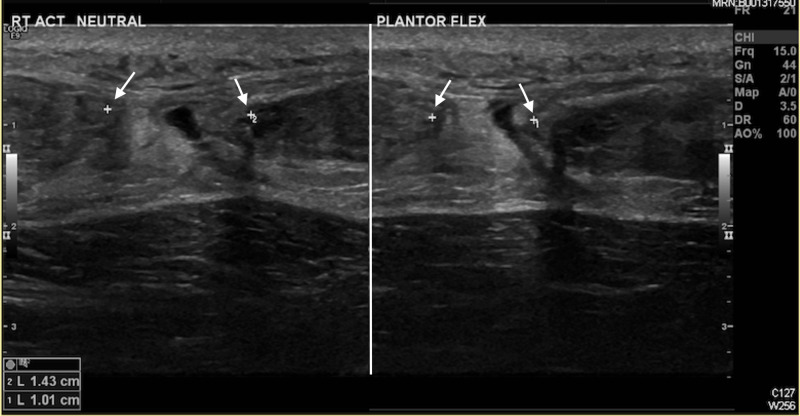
Ultrasound scan of a ruptured Achilles tendon measuring gap between ruptured ends (arrows) in both neutral position and plantar flexion.
